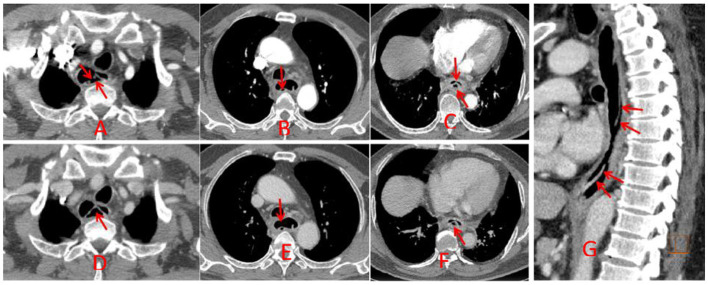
Contrast-enhanced CT in the axial (A–F) and sagittal (G) planes showed partial discontinuity and thickening of the posterior oesophageal wall (most pronounced in the lower oesophagus). A layered appearance was seen within the gas-containing submucosa. The lesion communicated locally with the oesophageal lumen and showed wall enhancement post-contrast. The peri-oesophageal space was ill-defined with exudative changes.
